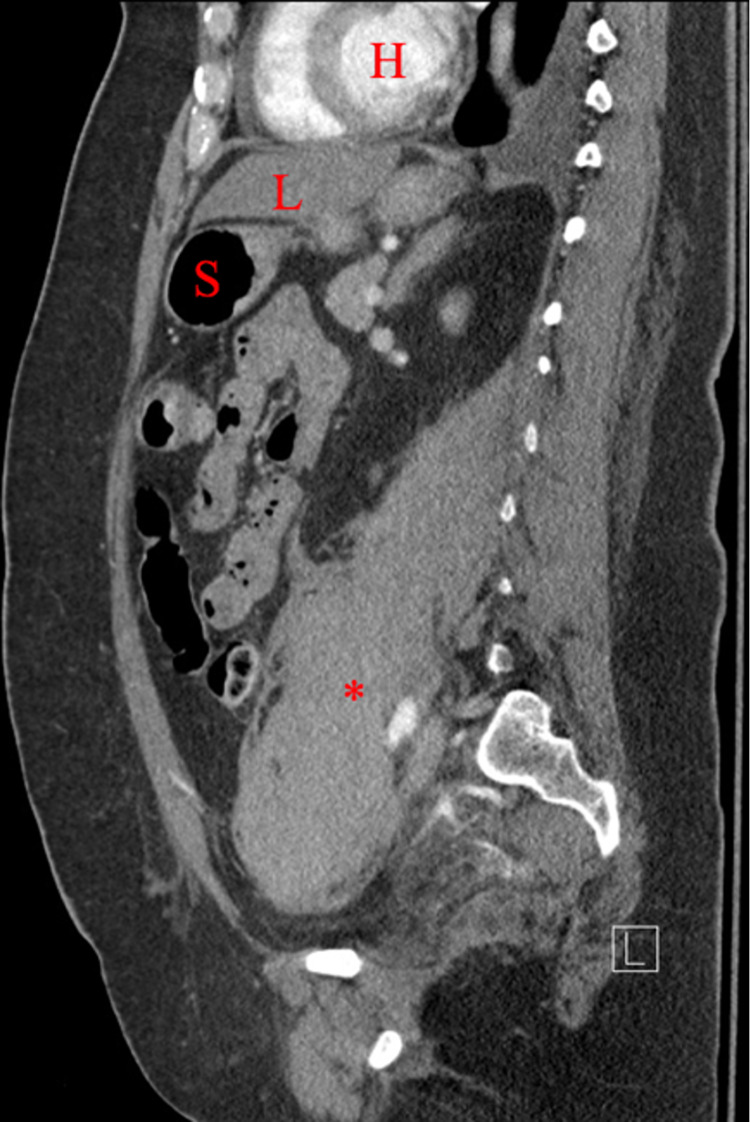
Sagittal CT demonstrating the retroperitoneal hematoma at the level of the sacrum, medial to the sacroiliac joint. H, heart; L, liver; S, stomach; *, retroperitoneal hematoma
